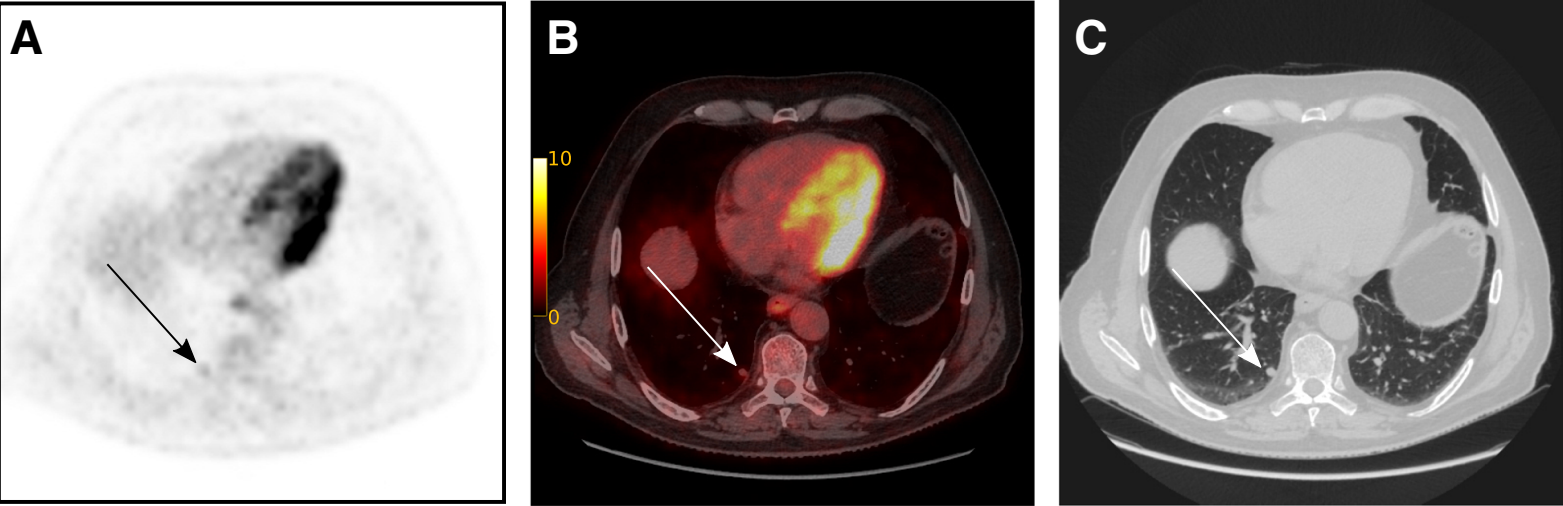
Solid subpleural nodule measuring 7 mm with increased metabolic activity (SUV max 2.8) in the right lower pulmonary lobe in the staging FDG-PET/CT. a FDG-PET; b FDG-PET/CT; c CT
